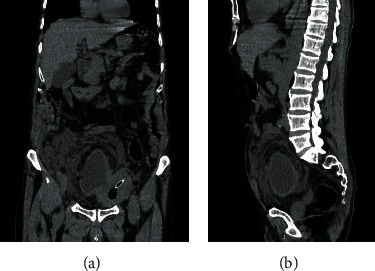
(a) Coronal reconstruction of the CT scan showing empty bladder with small amount of intra-abdominal fluid leaking from a ruptured large bladder diverticulum and perivesical fat infiltration. (b) Sagittal reconstruction of the CT scan showing a large full ruptured bladder diverticulum with fluid collection around it and ureteral dilation.
